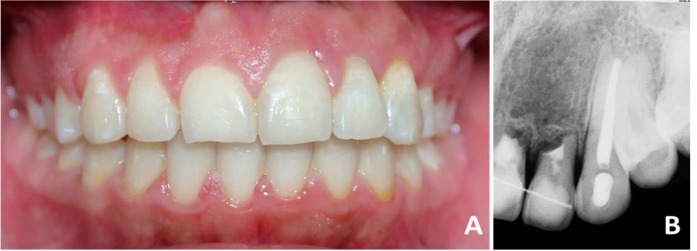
Braces removal and clinical-radiographic control after 2 years of surgical repositioning
